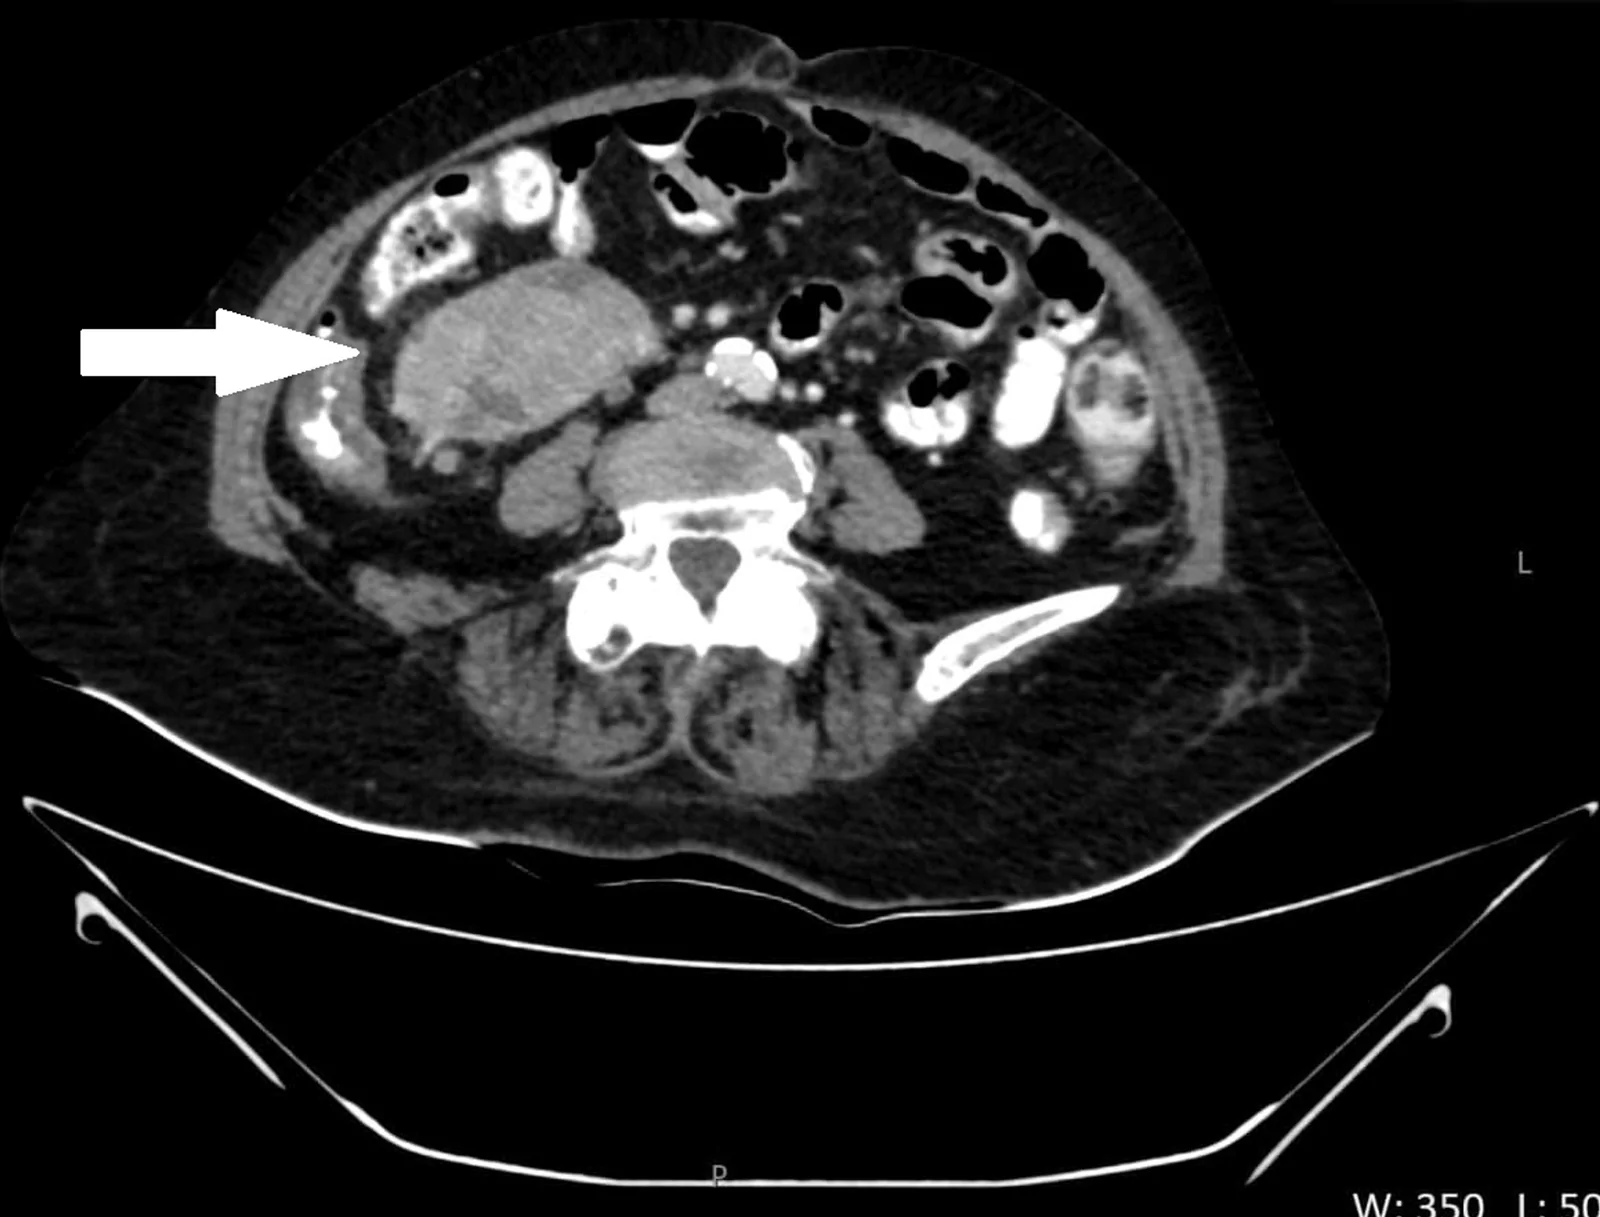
Contrast-enhanced computed tomography (CECT) Abdomen (axial view) Showing mesenteric mass in right iliac fossa.
